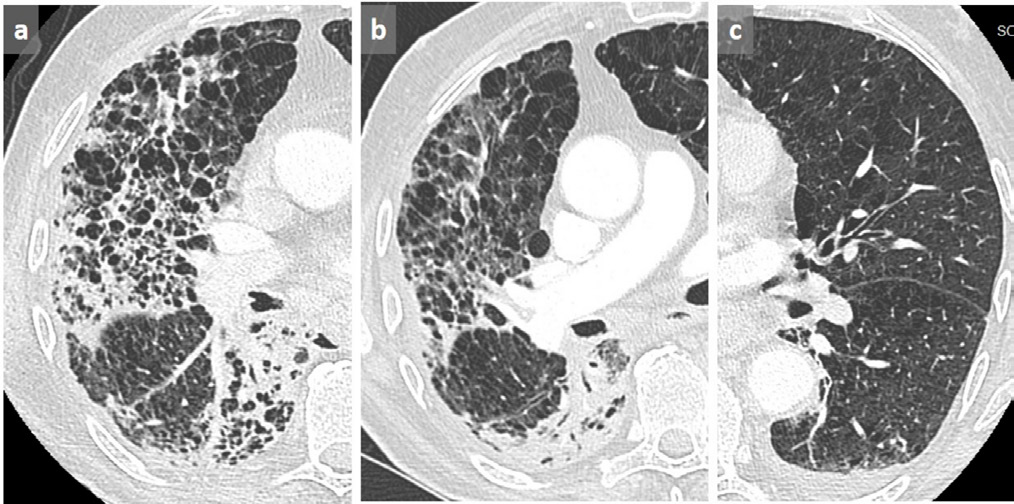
Pseudohoneycombing. Patient known for COPD presenting with cough. Honeycombing was reported on the first CT scan (a). Subsequent axial chest CT 2 months later showed disappearance of the cystic pattern that was related to a resolution of alveolar condensation after antibiotic therapy for *S.*
